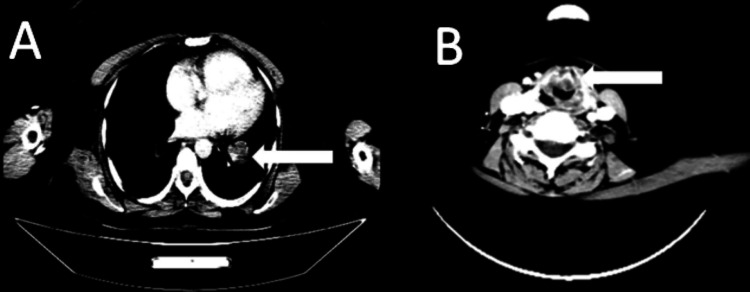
Horizontal CT scan image passing through the laryngeal tumor and the metastatic pulmonary lesion A) Infiltrative glottic laryngeal tumor process, 17 x 16 x 14 mm, affecting the anterior commissure and vocal cord. B) Proximal left lung secondary localization in the lower lobe, measuring 36 mm CT: computed tomography
